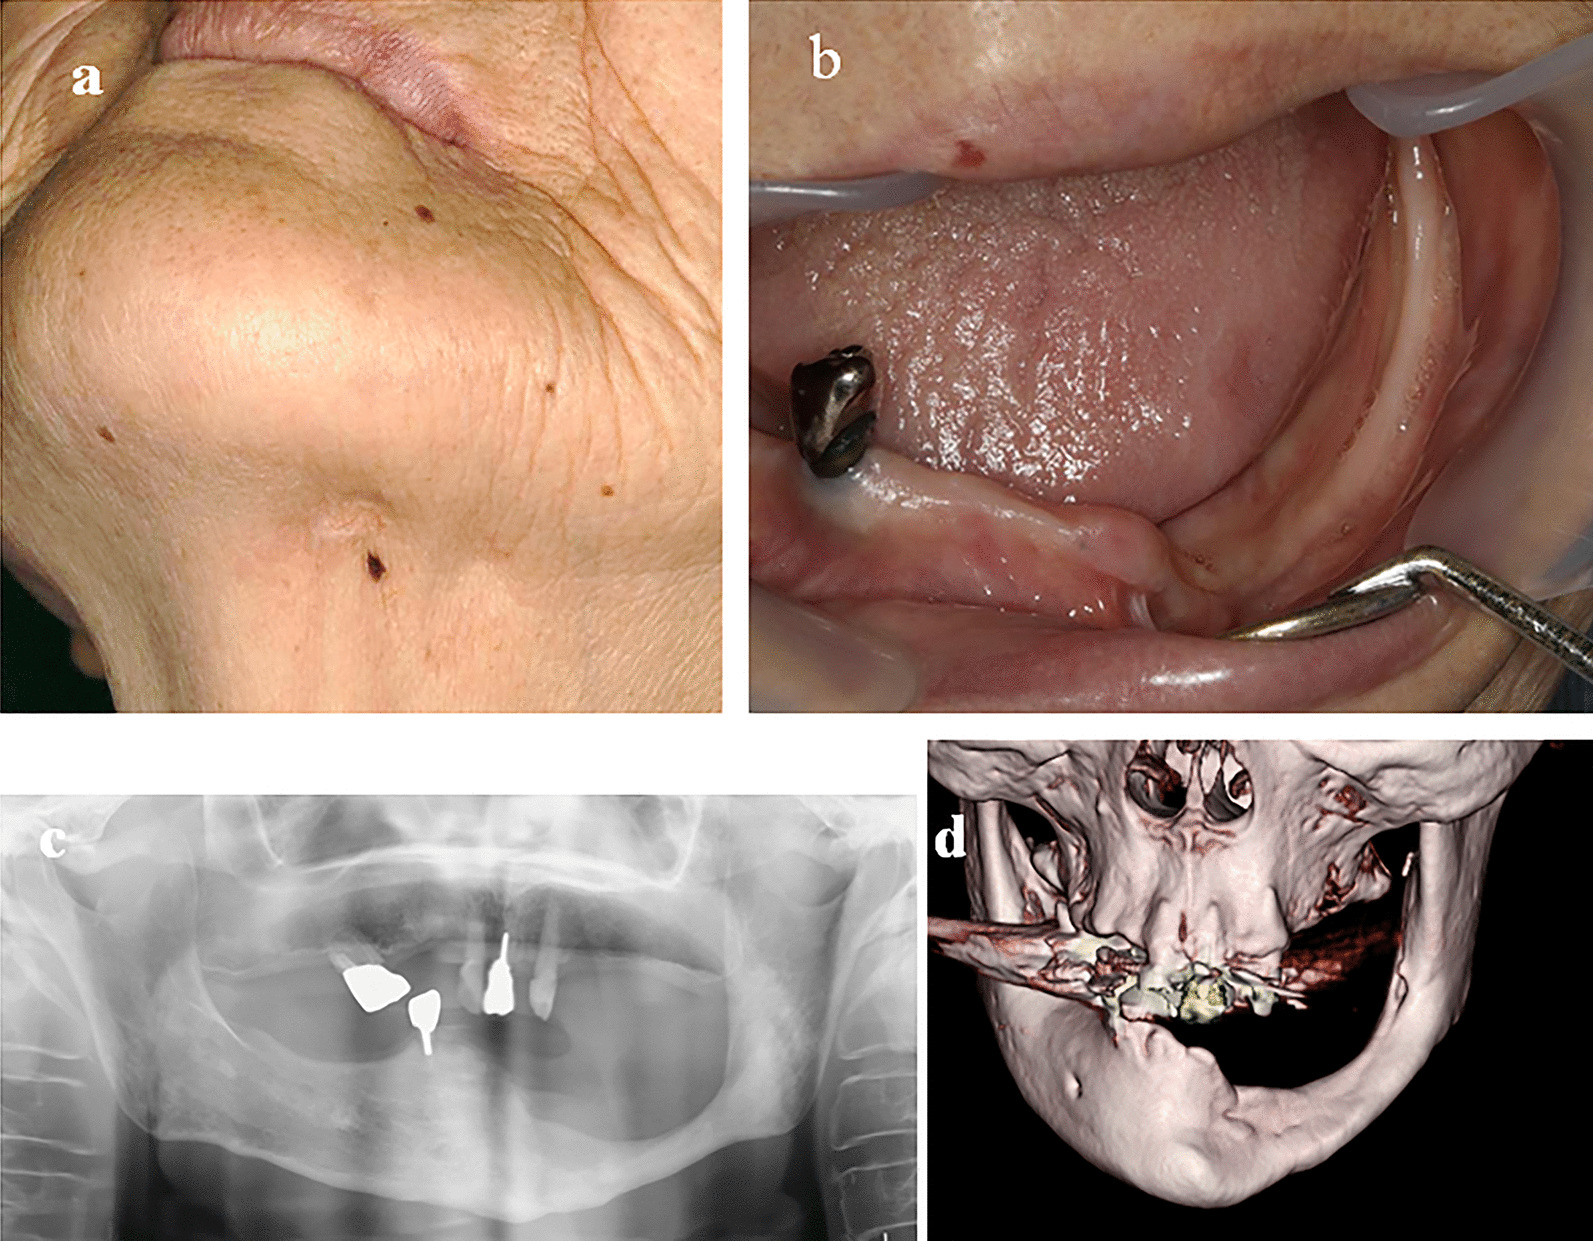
Appearance and imaging results of our patient 3 years after surgery. a Lower facial appearance showing with no inflammatory reaction or fistula. b Intraoral view showing no exposed bone. c Panoramic radiography showing regenerated bone of continuous thickness in the left mandible. d Three-dimensional computed tomography showing the preserved mandibular foramen and thick, continuous bone
